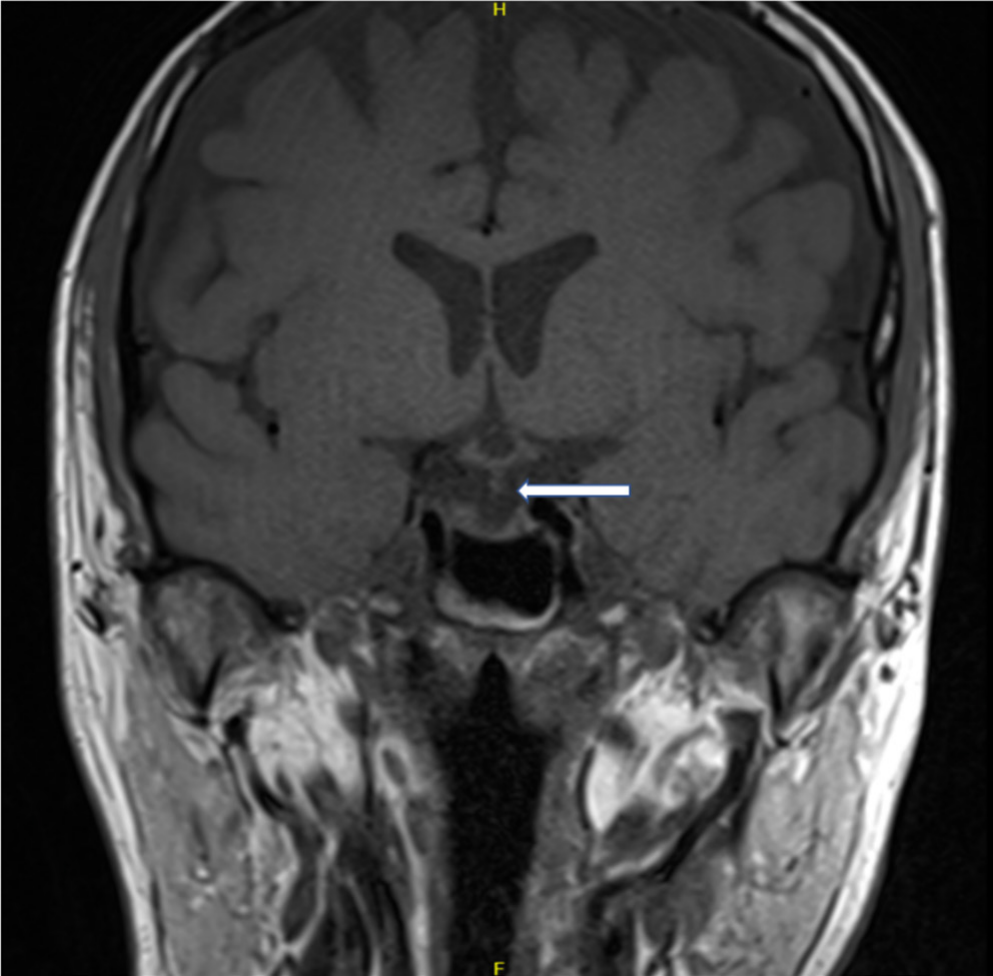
Coronal T1-weighted MRI imaging demonstrating the pituitary stalk which is normal in thickness, labelled with arrow.
